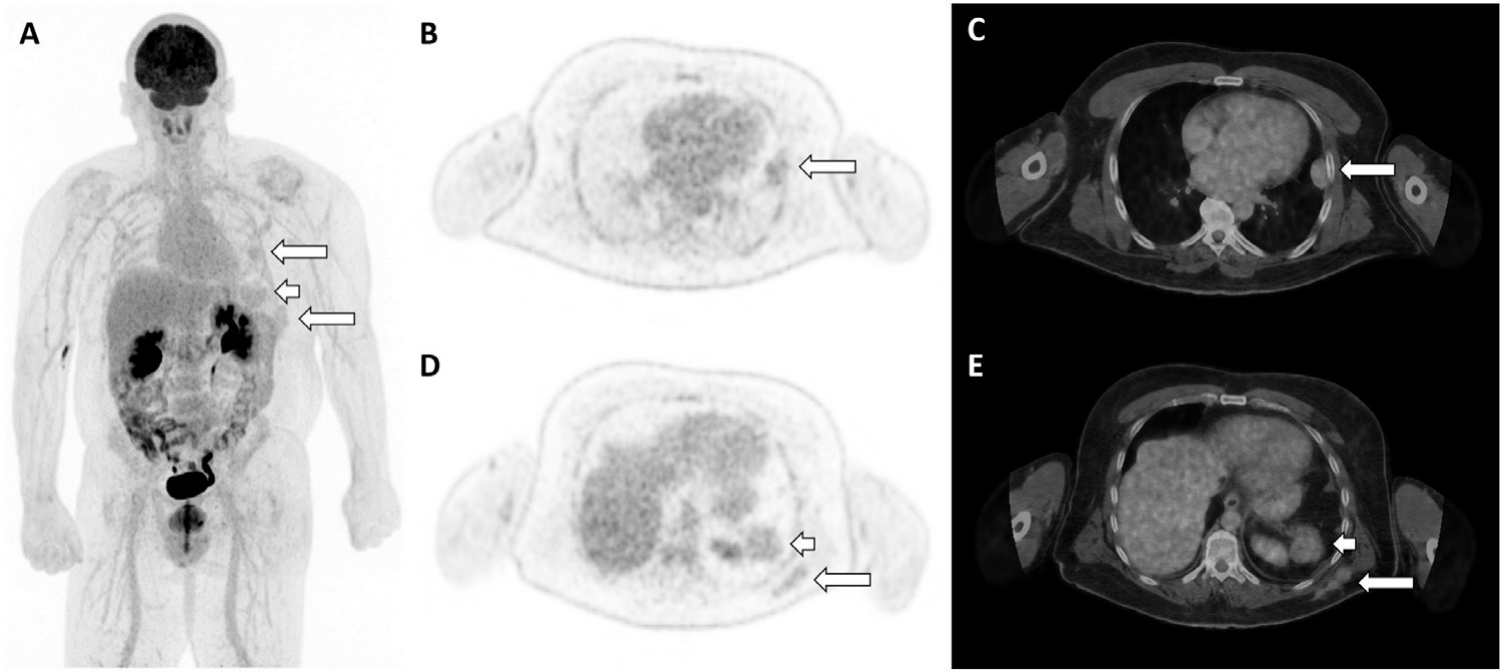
^18^F-FDG PET Maximal intensity projection (A), axial FDG PET (B and D) and axial hybrid imaging (C and E) demonstrated low-grade FDG avidity (SUVmax up to 2.6; long arrows) at the pleural and subcutaneous lesions, similar to background splenic activity (SUVmax 3.0; short arrows).
